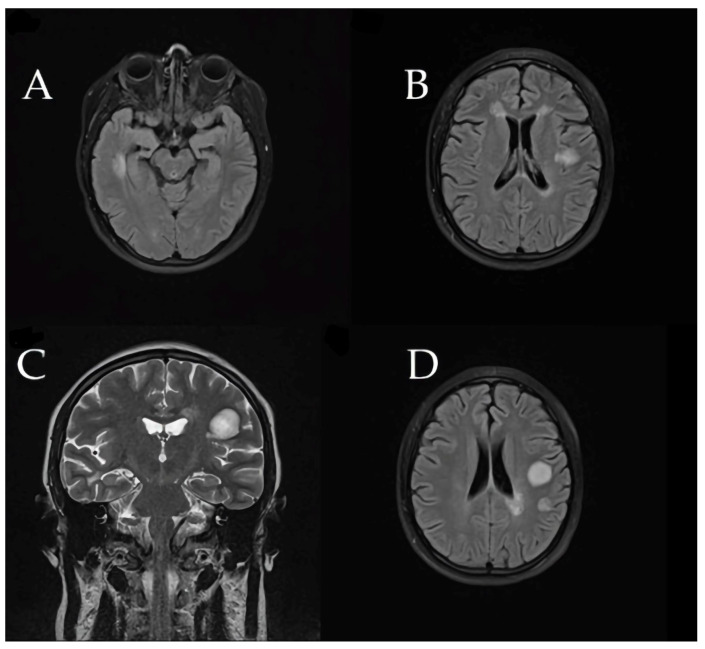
(A–D) Two years later, MRI reveals (A) Axial FLAIR sequence with marked regression in lesion size and surrounding edema compared to prior imaging. (B,D) Axial FLAIR sequences and (C) Coronal T2-weighted sequence identify multiple bilateral demyelinating lesions.
